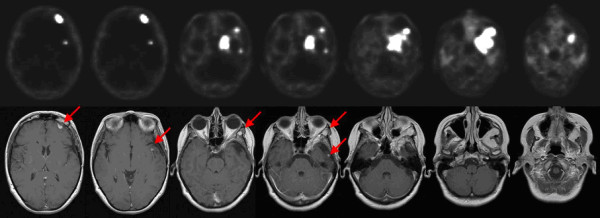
Multilocular relapse of a skull base meningioma 9 years after surgery in a 46-year old woman. 68-Ga-DOTATOC-PET (above) and T1 weighted MRI (below). Red arrows show distant lesions initially detected by PET imaging.
